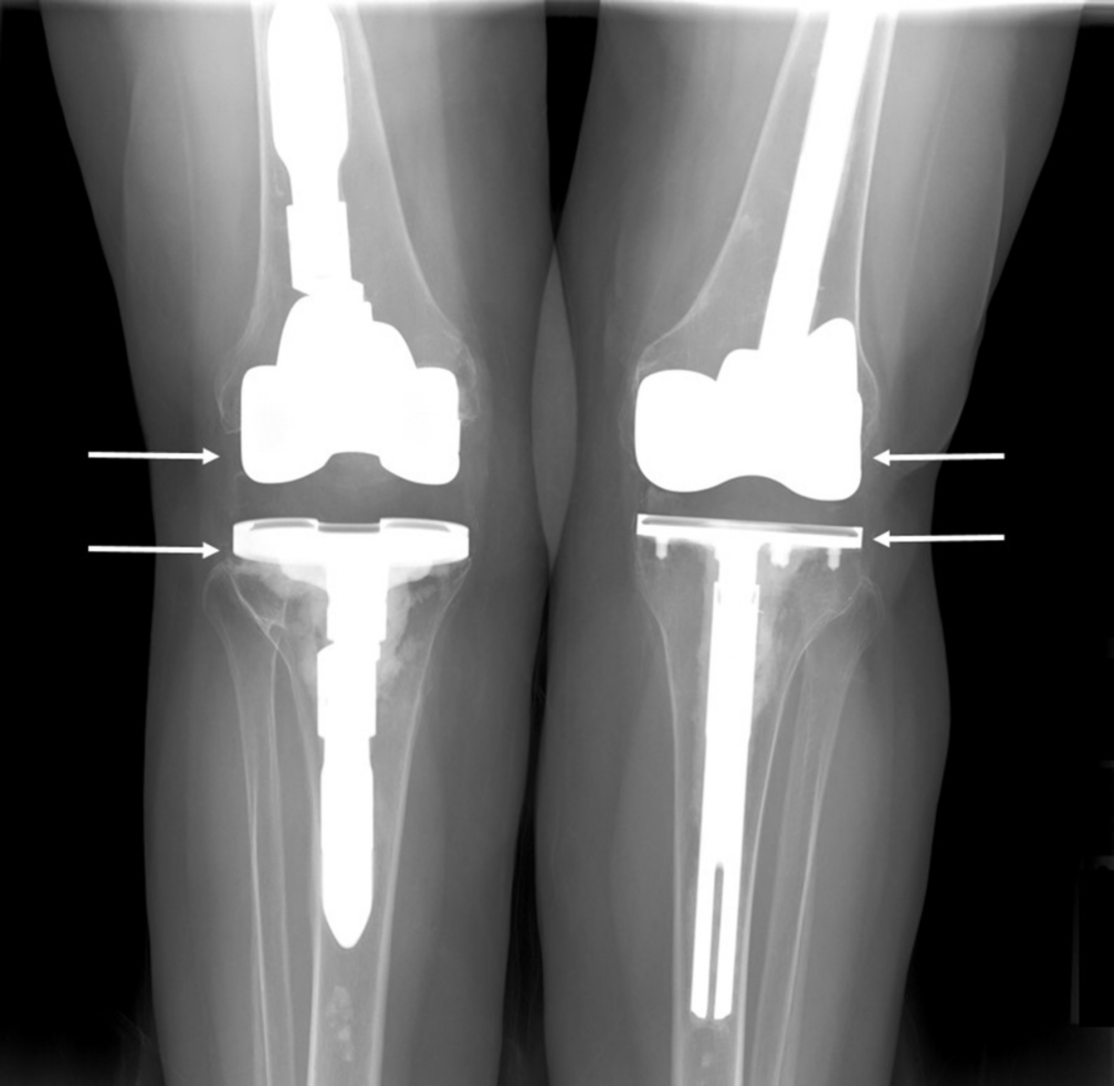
Preoperative X-ray showing hardware prior to bilateral revision total knee arthroplasty for instability (instability not visible on imaging)
